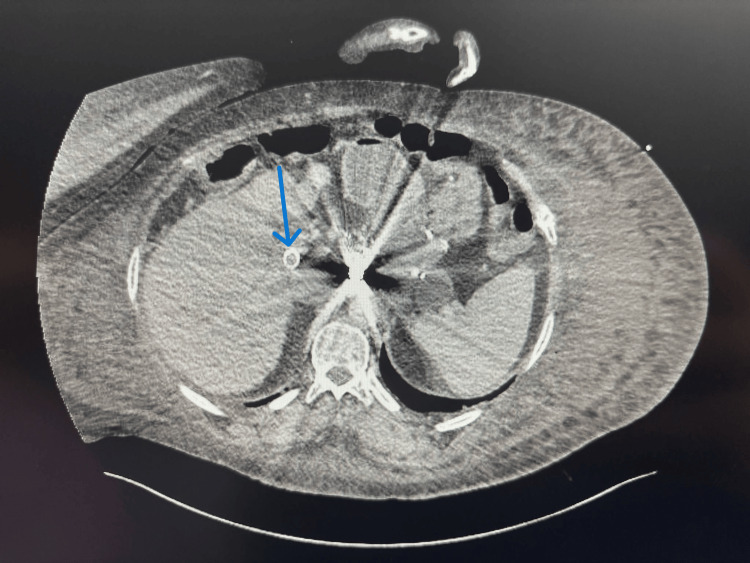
CTA abdomen - coronal view featuring patent TIPS CTA: computed tomography angiogram; TIPS: transjugular intrahepatic portosystemic shunt
